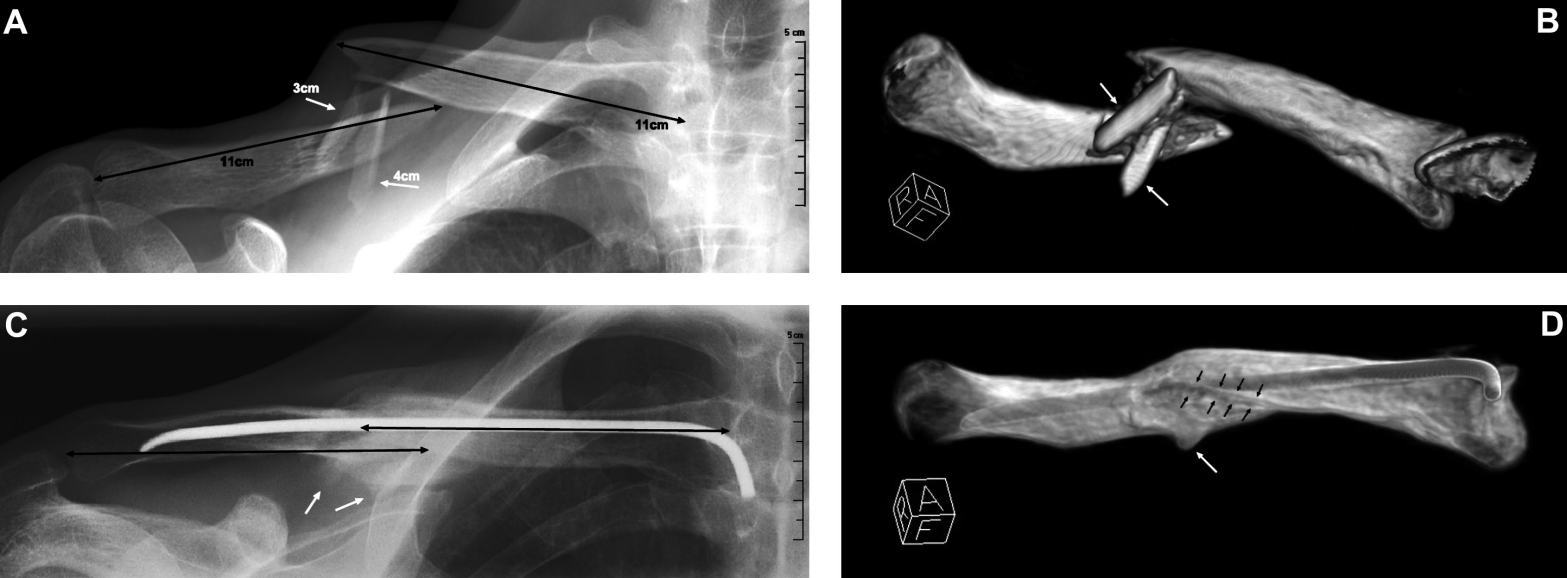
X-Ray and 3D reconstruction of the clavicle before and after operation. Panels A-D show pre and post operational images of the injured clavicle. A: X-ray from the displaced fracture two weeks after the accident. The black arrows indicate the two main fragments of the clavicle; the white arrows mark two additional fragments almost perpendicular to the clavicle main fragments heading towards the brachial plexus. The numbers indicate the approximate length of the fragments in cm. B: 3D reconstruction of a computer tomography done the day before the operation. The arrows indicate the fragments heading towards the brachial plexus. C: X-ray made the very first hour after the operation. The black arrows indicate the new configuration of the clavicle main fragments after open repositioning. The white arrows show the remaining fragments that could not have been removed during the operation. D: 3D reconstruction of a computer tomography done four months after the operation, before removing the nail. The black arrows show the line of consolidation and the white arrow shows the remaining residue of the fragment that could not have been removed but is almost resorbed now.
